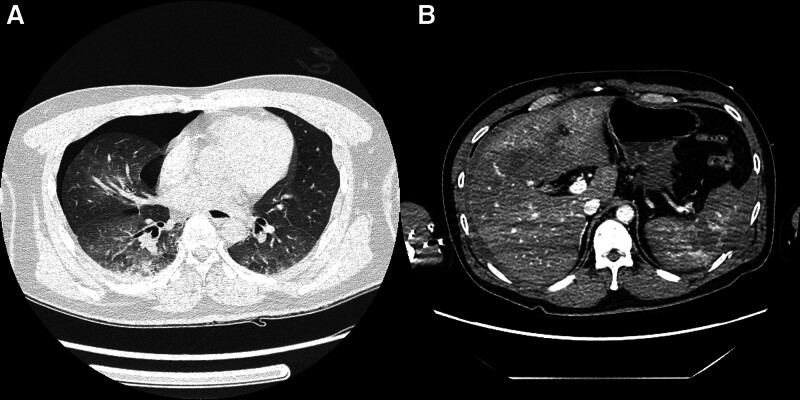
Abnormalities detected on the chest and abdominal computed tomography (CT) of the patient. (A) Posttraumatic chest computed tomography (CT) revealed a right-sided pneumothorax. (B) Grade III liver injury and Grade V splenic injury, accompanied by hemoperitoneum, were observed. CT = computed tomography.
